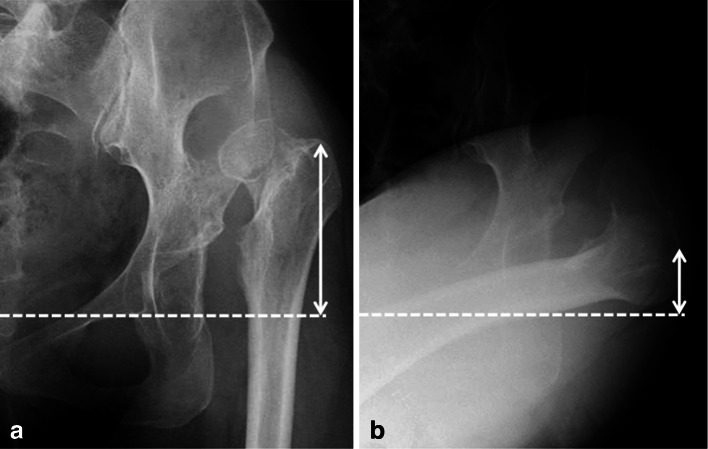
Preoperative anteroposterior radiographs showing the hip in a a neutral position and b a flexed adduction position. The *arrows* indicate the height of the greater trochanter, and the difference between these shows the expected descent of the tip of the greater trochanter. The *dotted line* indicates the teardrop line
